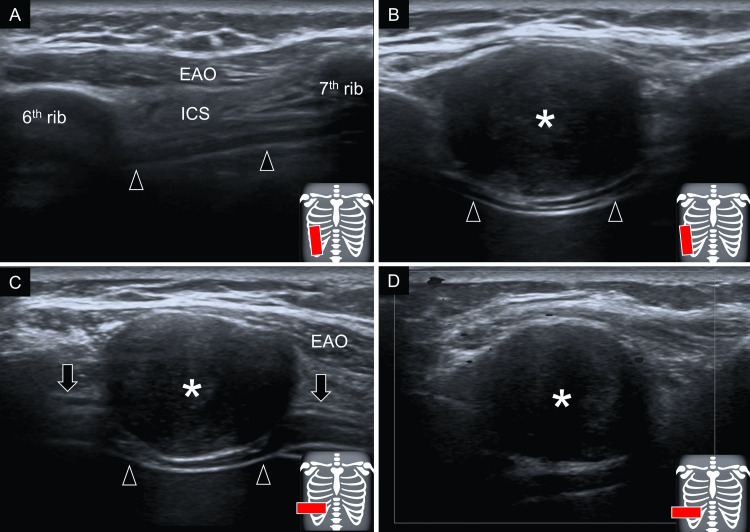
Ultrasound imaging of the intercostal space of the right lower ribs. The transducer is first placed over the right anterior lateral lower chest wall in the sagittal plane (A) and a mass (asterisks) emerged from the sixth intercostal space when relocating the transducer more laterally (B). The transducer was then pivoted to the horizontal plane to see the intercostal nerve (arrows) connecting to the mass (C). The power Doppler imaging showed a petechial increase of vascularity (D). (Arrowheads showing pleura and red square showing the footprint of the transducer.) EAO: external abdominal oblique muscle; ICS: intercostal muscle
